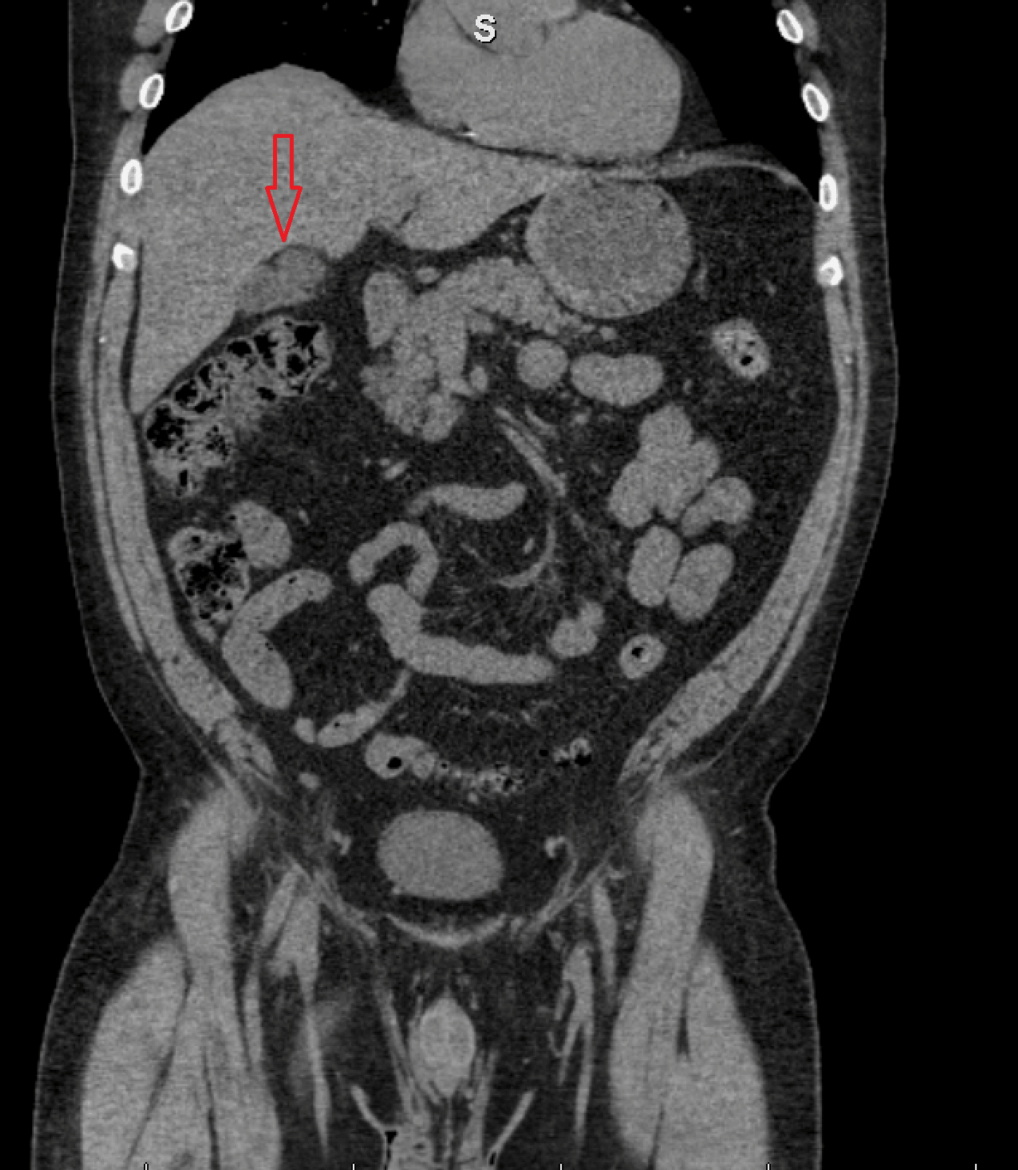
Abdominal pelvic CT without contrast showing gallbladder wall thickening.
